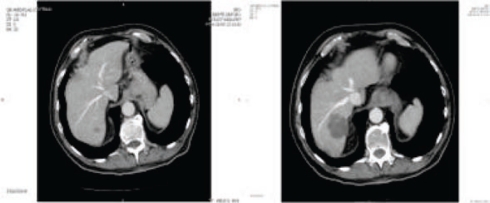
Liver metastases from CRC in segment 7: (a) CT before treatment; (b) post-treatment CT shows a low-density area (= necrosis) where thermal ablation has been performed
